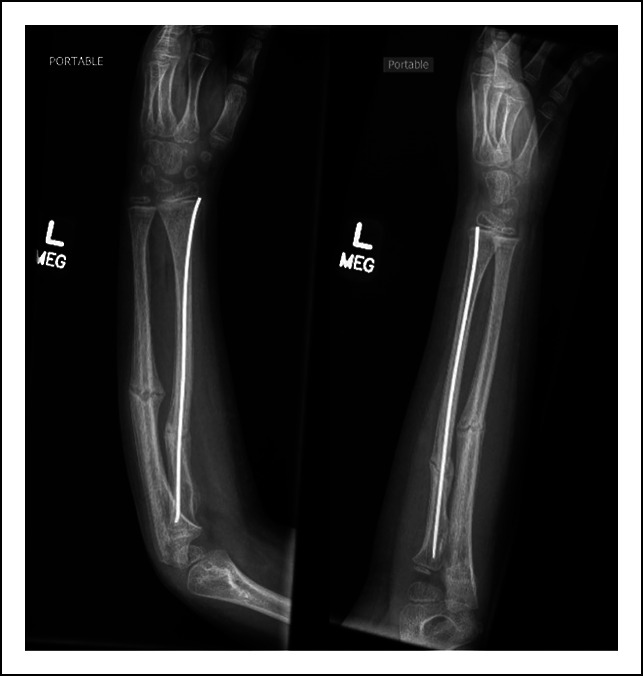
Radiographs (anteroposterior and lateral views) at the 2-week follow-up appointment after ulnar rod removal.
